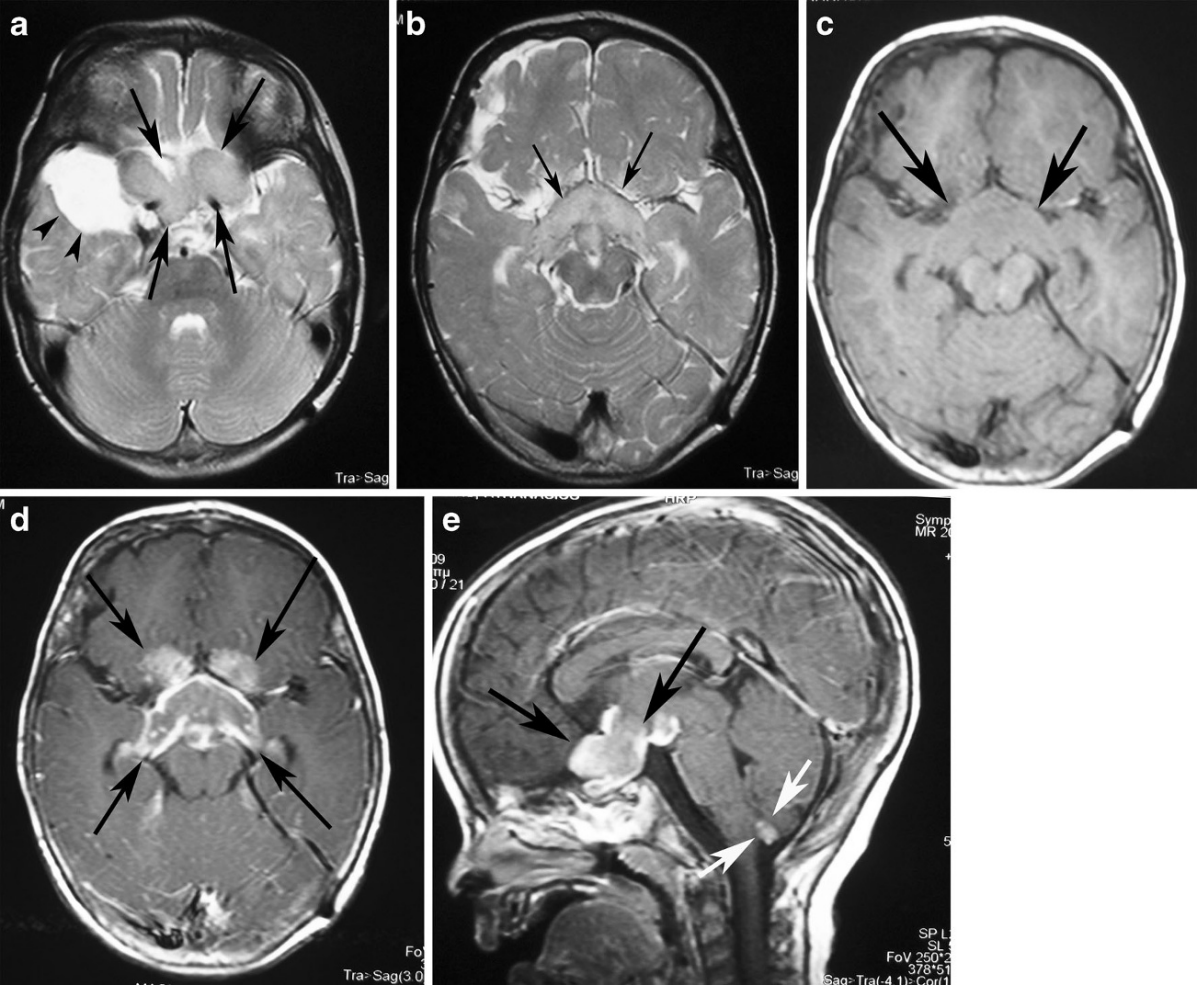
A pilocytic astrocytoma involving optic pathways. a An axial T2-weighted image shows a large mass originating in the region of the optic chiasm/hypothalamus (arrows). Note the arachnoid cyst in the right middle cranial fossa (arrowheads). b An axial T2-weighted image in a higher level shows the hyperintense tumour extending to the optic radiations (arrows). On this T1-weighted image (c), the mass is isointense compared with grey matter. T1-weighted axial (d) and sagittal (e) post-contrast images show heterogeneous enhancement extending anteriorly towards the optic nerves and posteriorly towards the optic tracts. Note the enhancing nodule dorsal to the medulla due to dissemination of the pilocytic astrocytoma (arrows)
